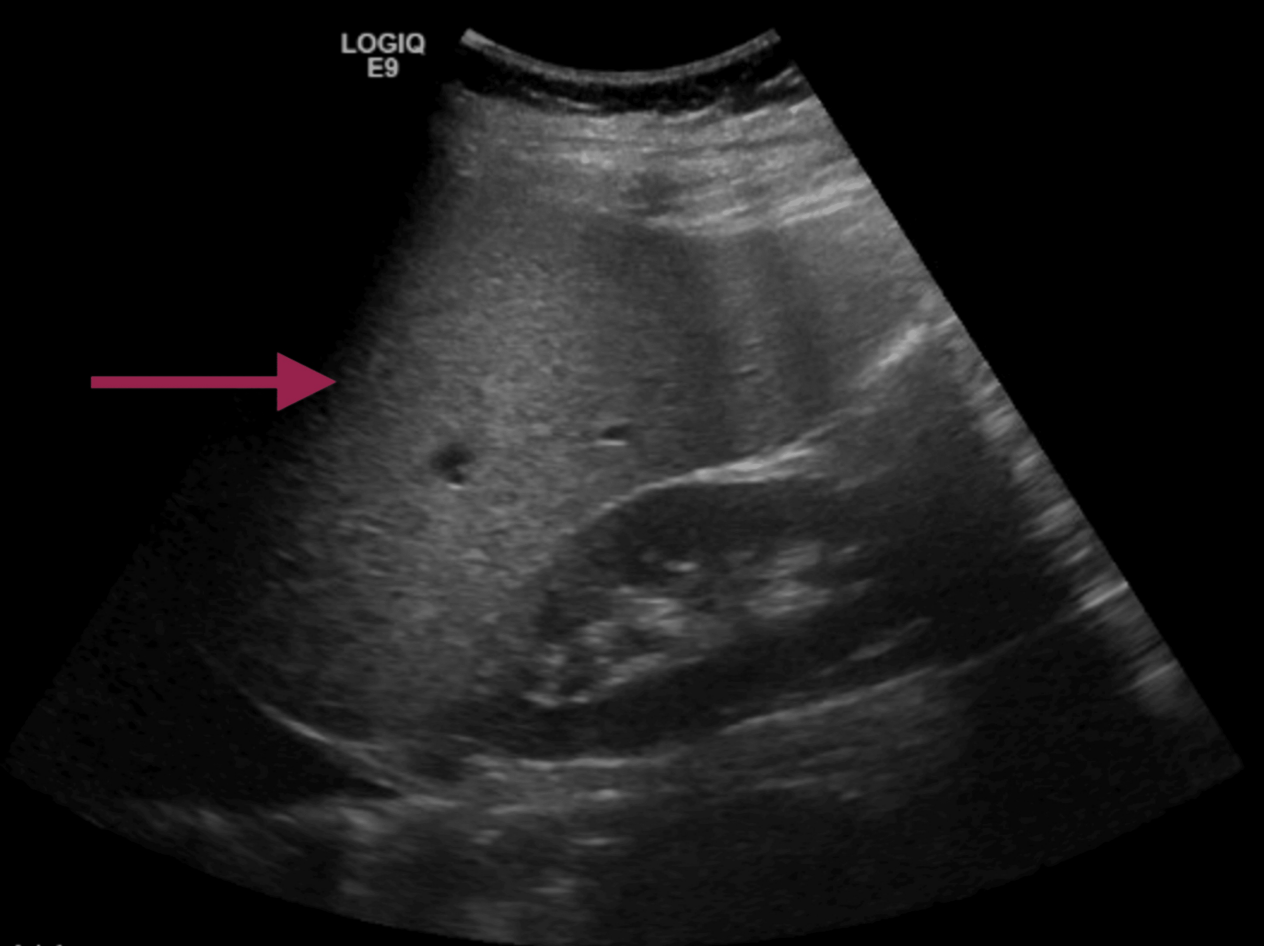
Abdominal ultrasound performed in the emergency room The image revealed homogeneous liver parenchyma with diffuse infiltrative steatosis (arrow)
